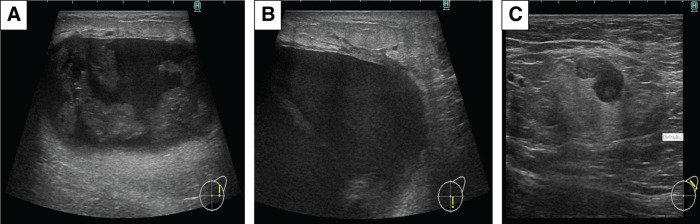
US after PEM+AC, 2nd cycle, at the time the patient reported rapid tumor growth and pain (A: inside the left external superior portion of the tumor; B: edematous changes in the subcutaneous tissue; C: axillary lymph node). These findings suggest inflammatory changes in the breast tumor. PEM+AC, doxorubicin+cyclophosphamide+pembrolizumab; US, ultrasonography
